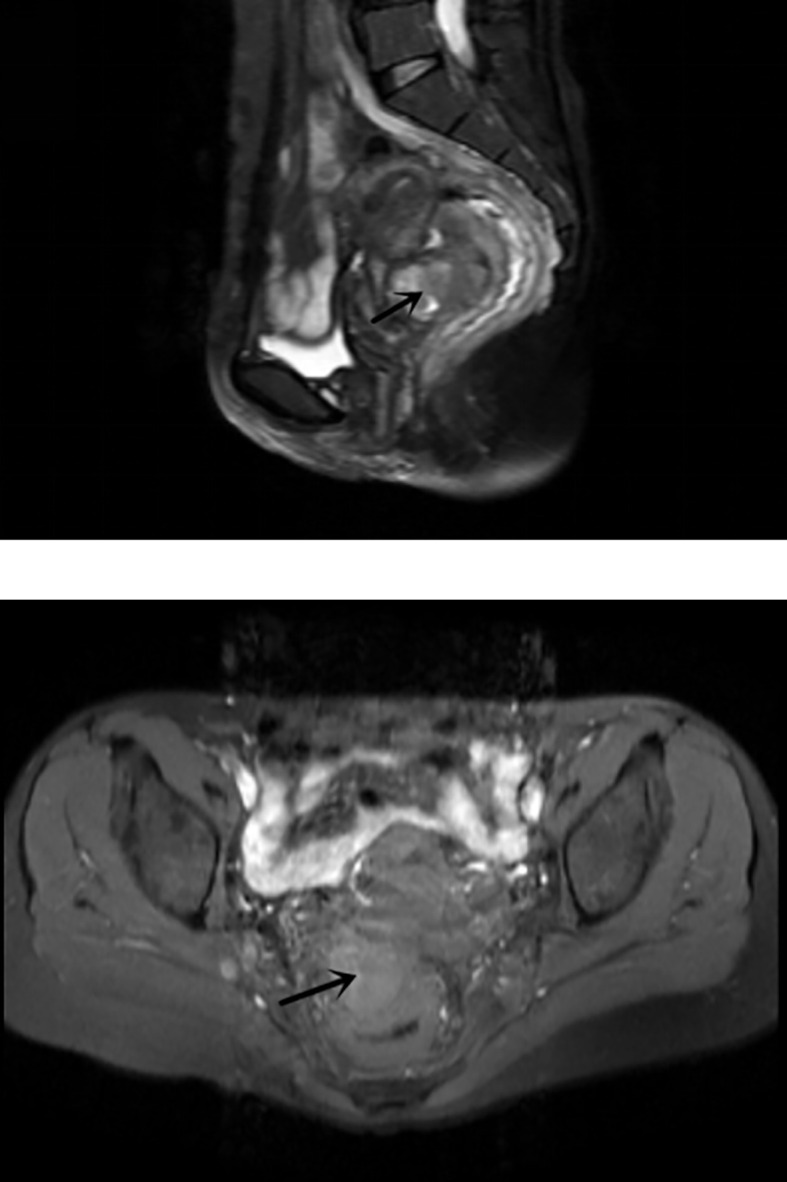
Pelvic MRI: Hybrid signal packet block at the right rear of the uterus.
